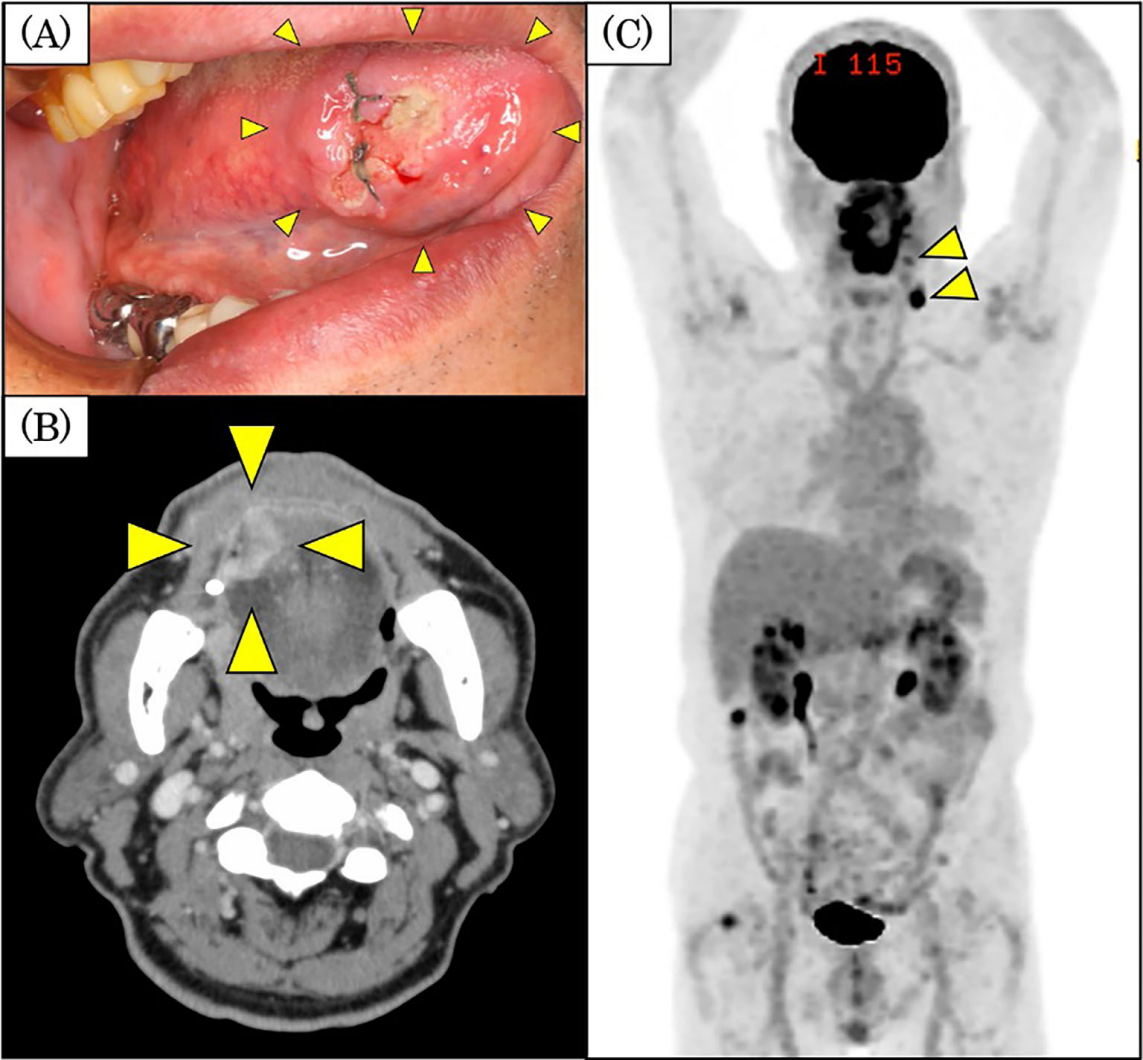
Findings of the tongue tumor and imaging findings. Intra‐oral findings (A). A tumor is visible on the right side of the tongue. The tumor was 28 mm in its longest diameter, with induration around the tumor and in the deep tissues. CT findings (B). The tumor measured 24.0 × 19.3 × 14.2 mm from the right lingual apex to the edge of the tongue. FDG‐PET/CT findings (C). The cervical lymph nodes are visible at left levels IB and III (arrowheads), with abnormal accumulation and SUV_max_ values of 4.10 and 9.00, respectively. There was no uptake in the lymph nodes in the right neck. CT, computed tomography; FDG‐PET/CT, fluorodeoxyglucose‐positron emission tomography/CT; SUV_max_, standardized maximum uptake value.
